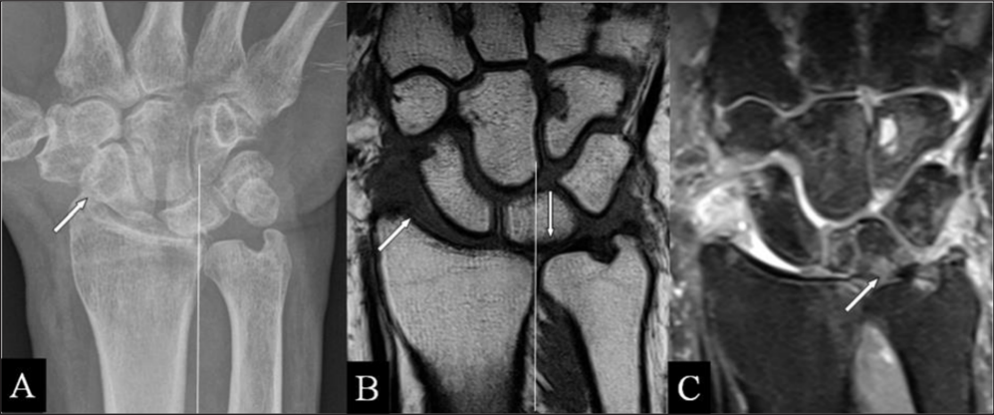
Ulnar translation with abutment. (A) PA plain radiograph; (B) Coronal SE T1-WI; and (C) Coronal SE PD-WI FS. (A–C) Lateral widening of the radioscaphoid joint (oblique arrow) and the lunate bone overlapping less than 50% with the corresponding radial articular fossa due to the ulnar translation of the carpus. (B, C) Cartilage destruction, oedema, and an accompanying lesion of the TFCC.
